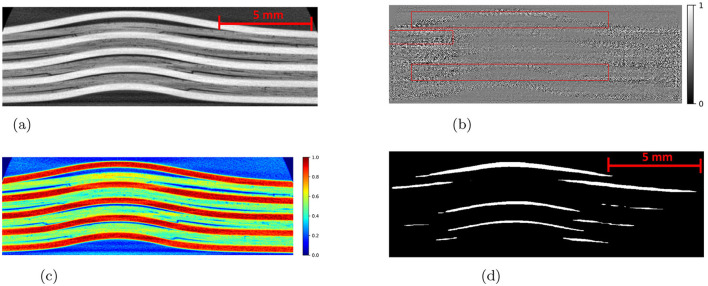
Visualization of IG results for impact energy of 12.5 Joules. (c) Shows the attribution map produced using the IG method with the modified loss function applied on the trained Mask R-CNN model, using the CT slice of the FML plate shown in (a). Example red boxes shown in attribution map (b) highlight attribution of damages. (a) CT slice with impact energy of 12.5 Joules. (b) Attribution map overlay. (c) Attribution map (modified loss). (d) Predicted mask.
